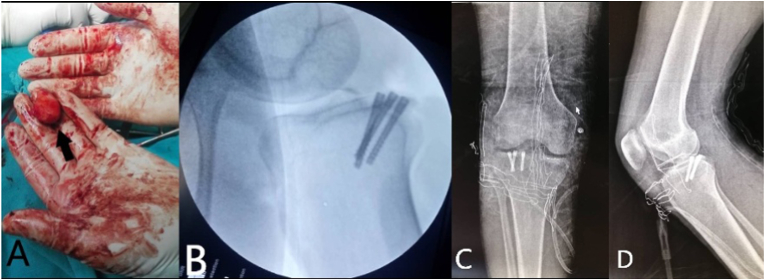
Intraoperative images; (A); a large free fragment (3*2 cm) on the surgeon's hand after extraction (black arrow), (B); the free fragment fixation with Herbert screws during the surgery. Immediately postoperative images; Anteroposterior (A) and lateral (B) X-rays show the fragment repositioning and fixed with three Herbert screws.
